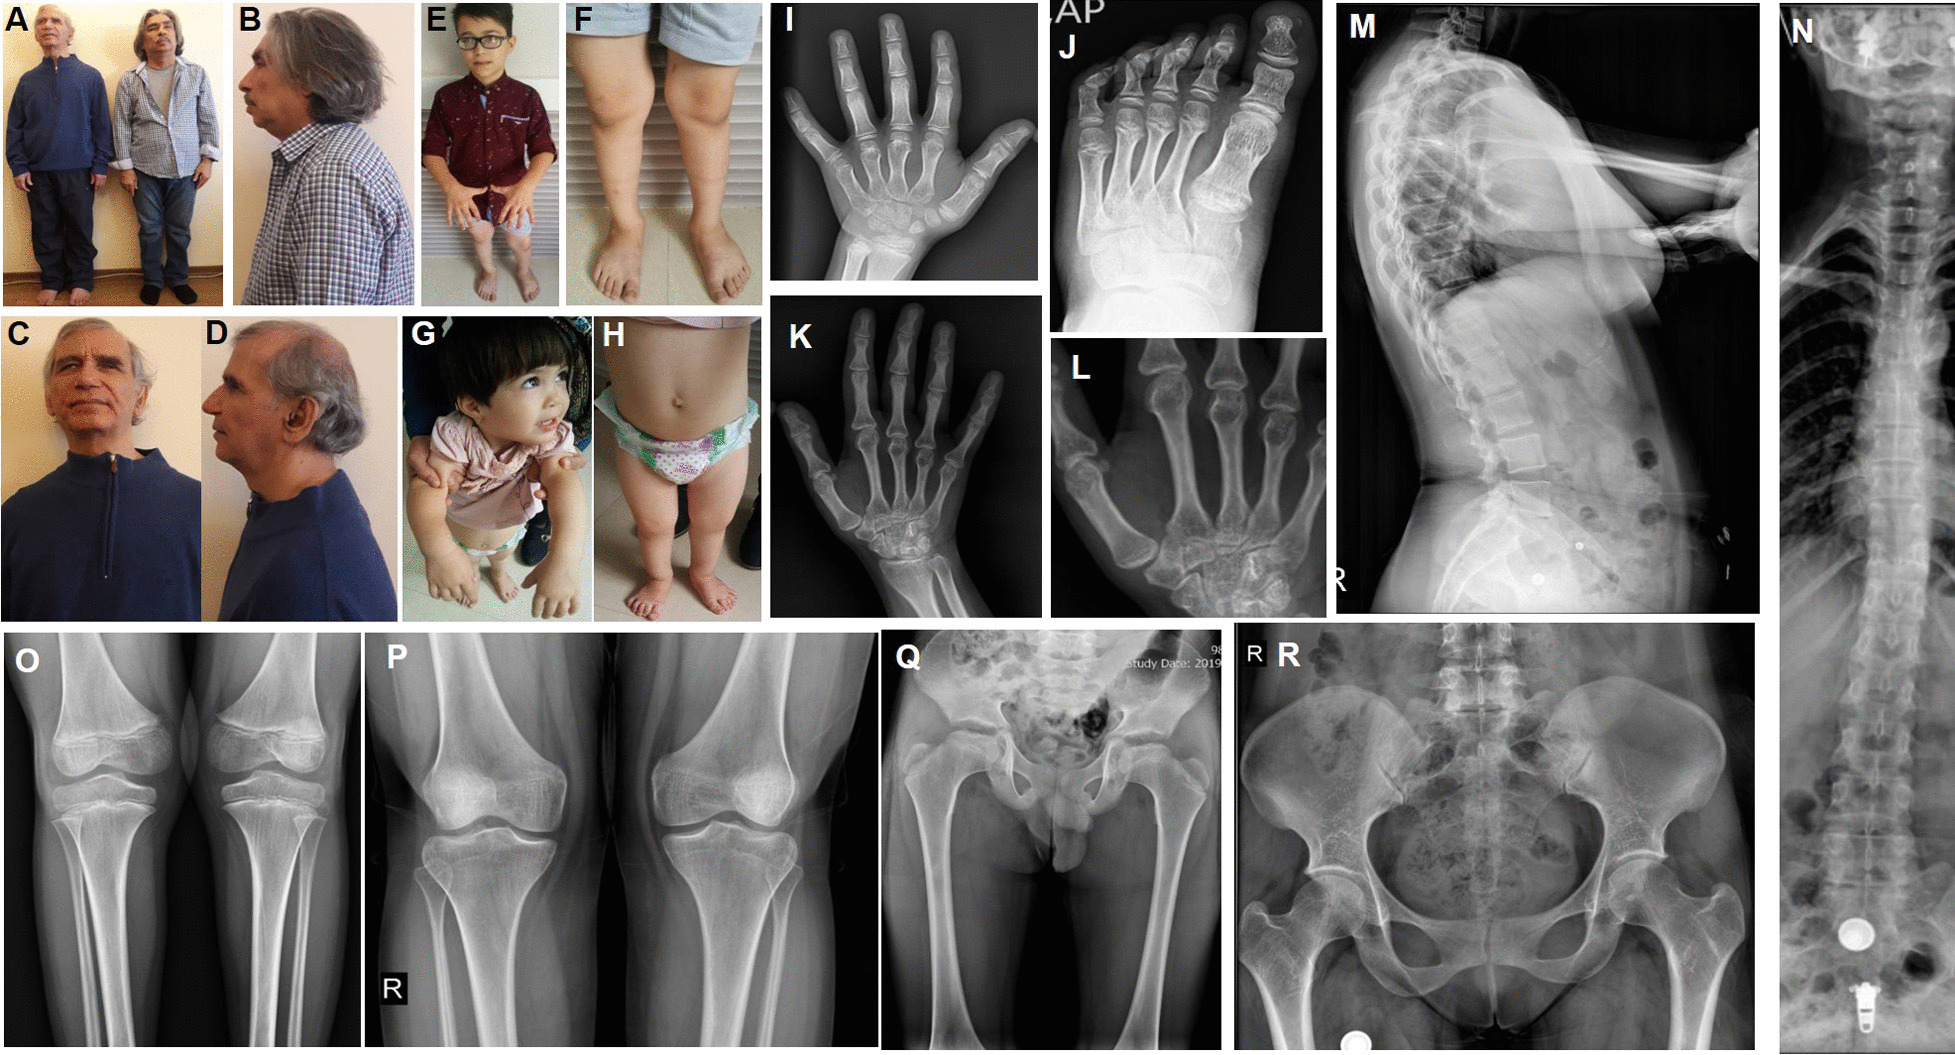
Pictures of four affected individuals and standard radiographs of the spine, pelvis and limbs of two patients. A–D Pictures of two affected individuals from family 2 who both have herniated cervical discs. E, F Pictures of affected individual II1 from family 3 showing short stature, pes planus, bowed tibia, genu valga and rotated distal femura distal femur. G, H Clinical appearance of proband II1 from family 1 showing pes planus, mild midface hypoplasia, upturned nose and low set ears. I, J Hand and foot radiographs showing short metacarpalia and a broad big toe for individual II1 from family 3 at the age of 10 years. K, L Radiograph images of the right hand and wrist joint of individual II1 from family 1 at the age of 28 years, showing short metacarpalia with widened epiphyses and an irregular radius epiphysis. M, N Radiographs of the spine of II1 from family 1, showing mild platyspondyly of the thoracic spine as well as signs of ankylosing spondylitis. O Knee radiograph of proband II1 from family 3 showing genua valga and irregular femur epiphyses. P Knee radiograph of proband II1 from family 1 demonstrating genua valga and widened femur epiphyses. Q Pelvis radiograph individual II1 of from family 3 showing a flat acetabular roof with irregularities and flattened capiti femori, as well as broadened and shortened necks. R Radiograph of the pelvis of individual II1 from family 1 showing a relatively narrow intraarticular space but well developed capiti femori and no flattening of the acetabular roof
